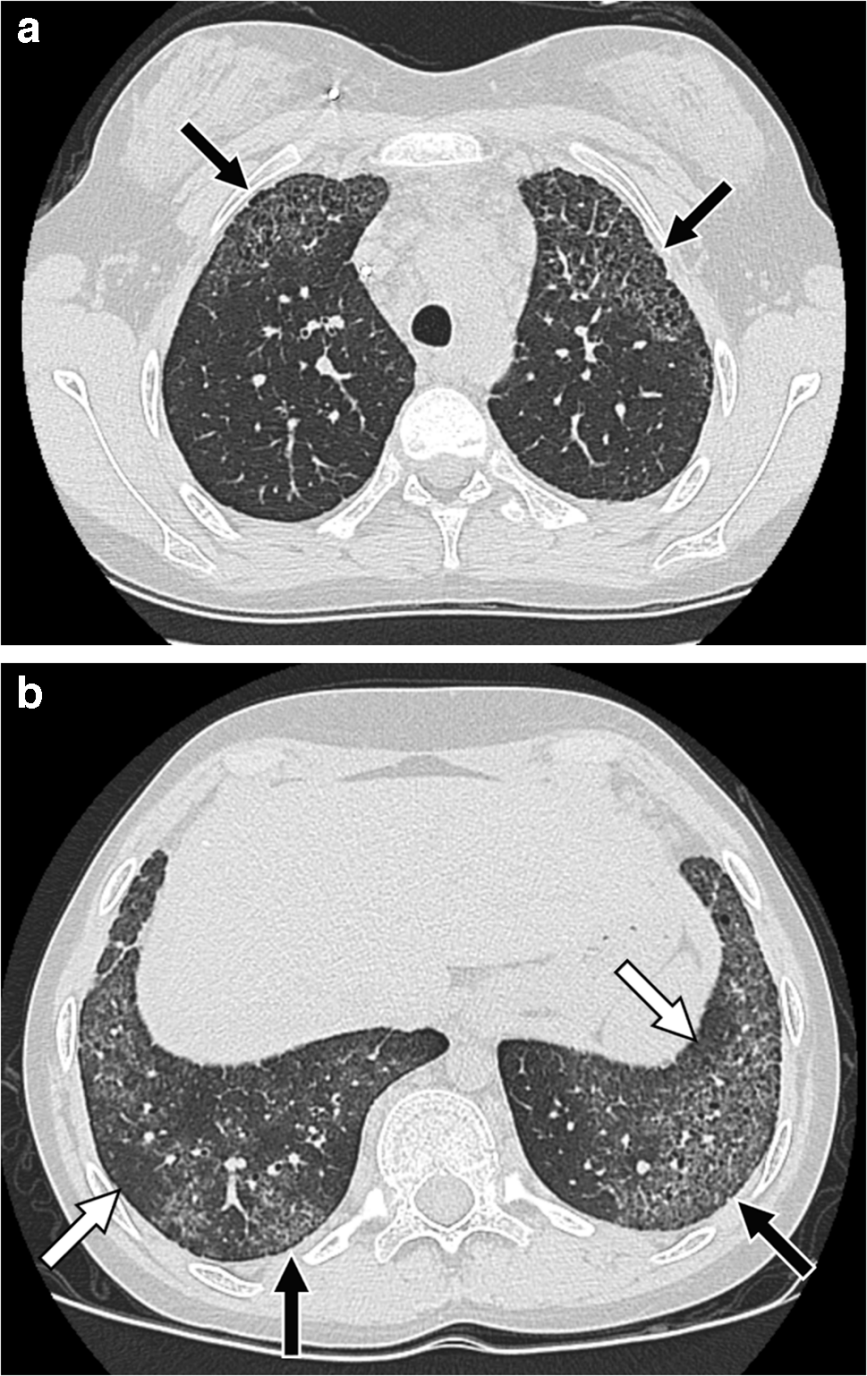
13-year-old female with mixed connective tissue disease. a, b Axial CT images without intravenous contrast showing ground-glass opacity, reticulation, and bronchiolectasis in the lower lobes and anterior upper lobes with areas of subpleural sparing in the lower lobes (black arrows) suggesting nonspecific interstitial pneumonia
